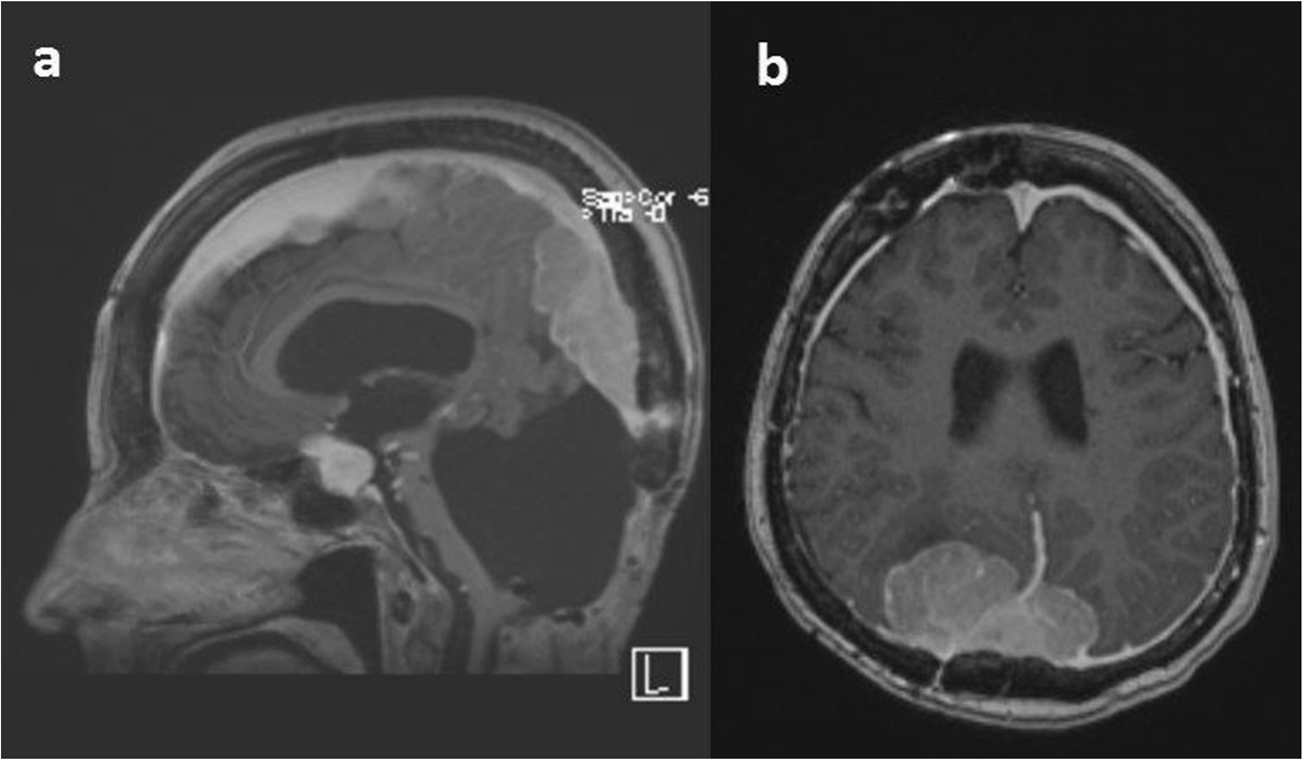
Sagittal MRI showing meningioma along the falx cerebri and the sella region, but no residual tumor in the posterior fossa (2011)
